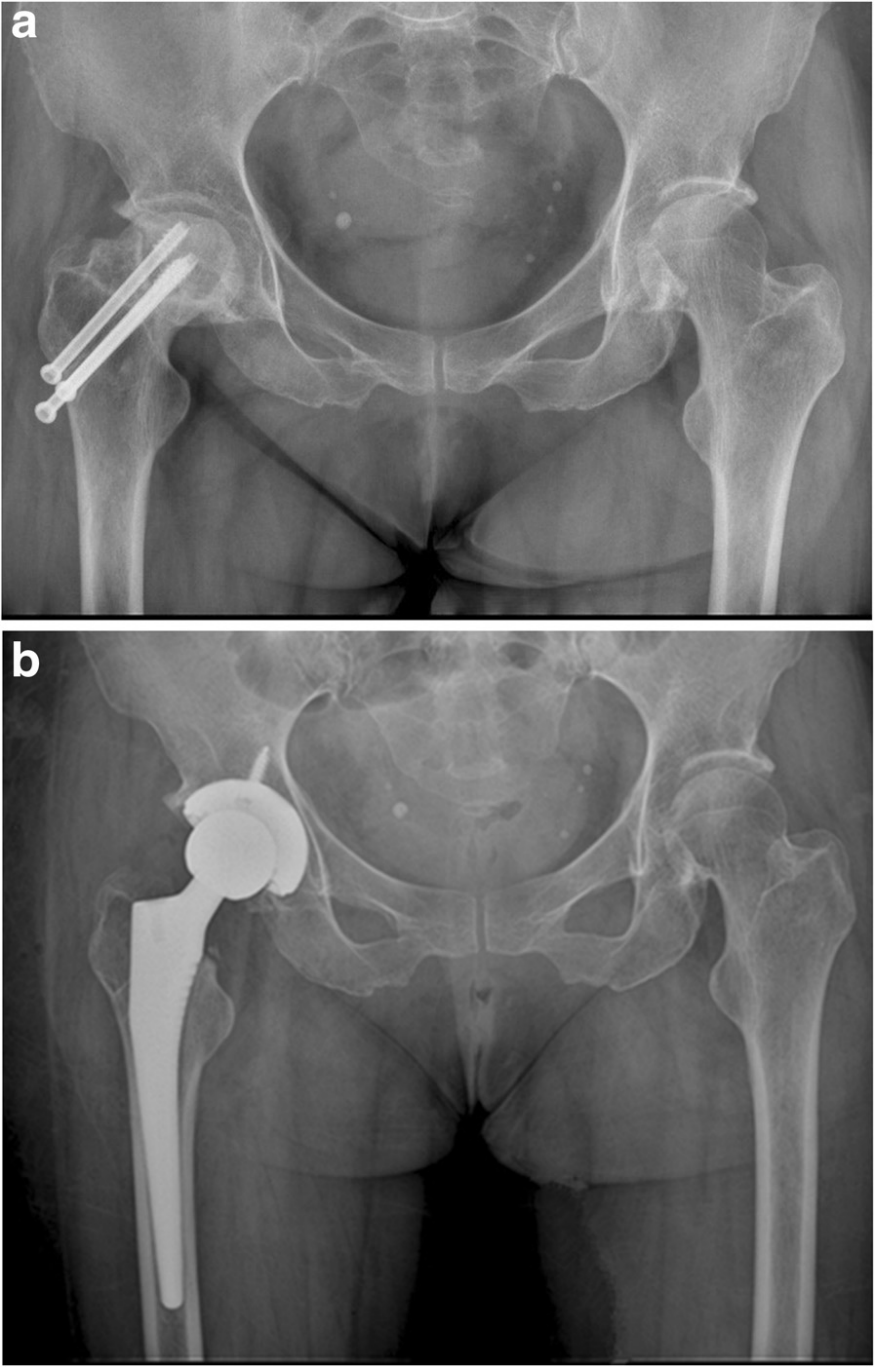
a A preoperative radiograph of the hip of a 72-year-old woman who had femoral head osteonecrosis due to femoral neck fracture. b A radiograph at 42 months after implantation of conventional cementless femoral stem showed solid fixation in a satisfactory position
